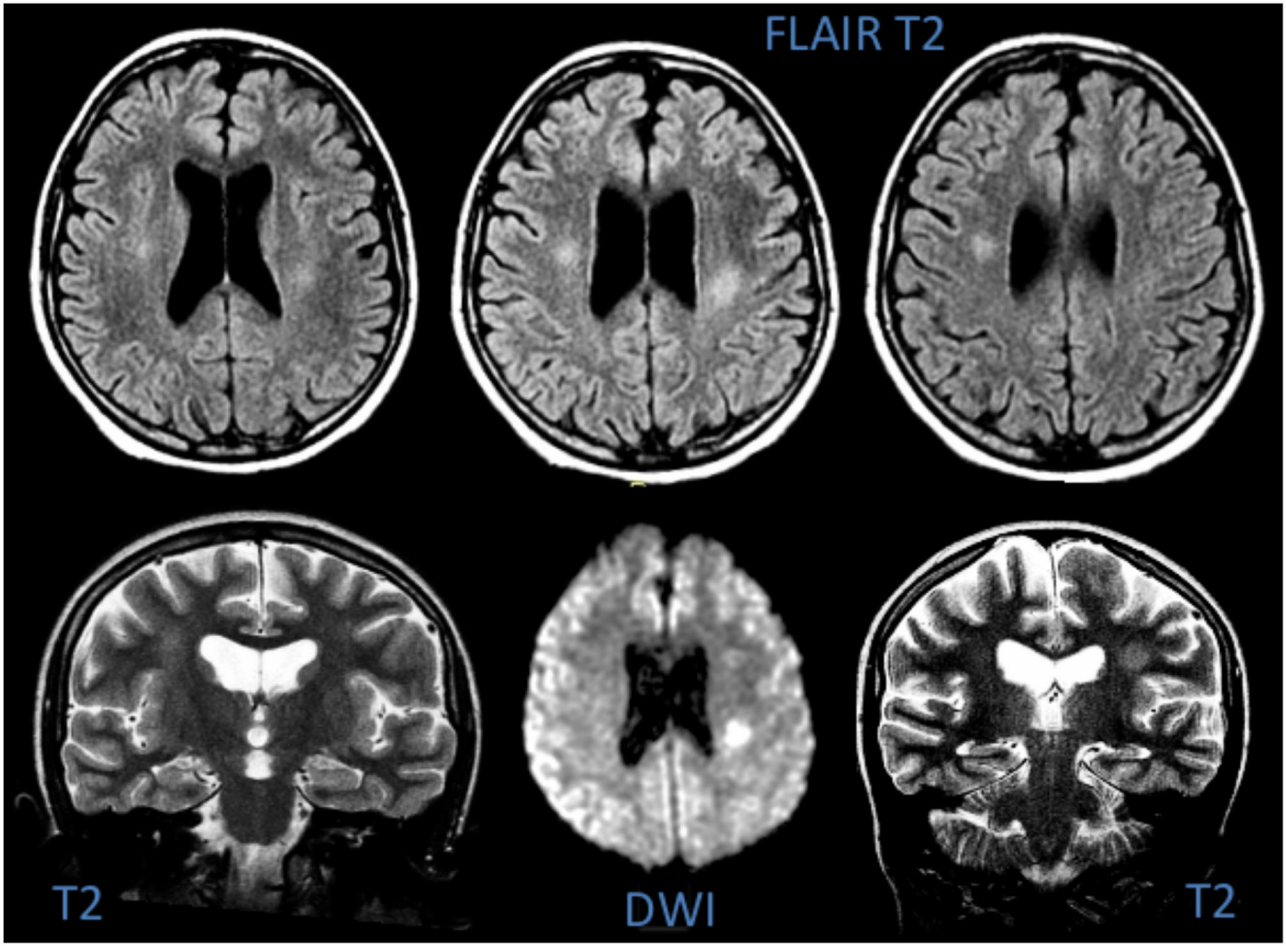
MRI of Patient 6, showing hyperintensity of the deep white matter on T2-weighted images and signs of cytotoxic oedema in DWI.
